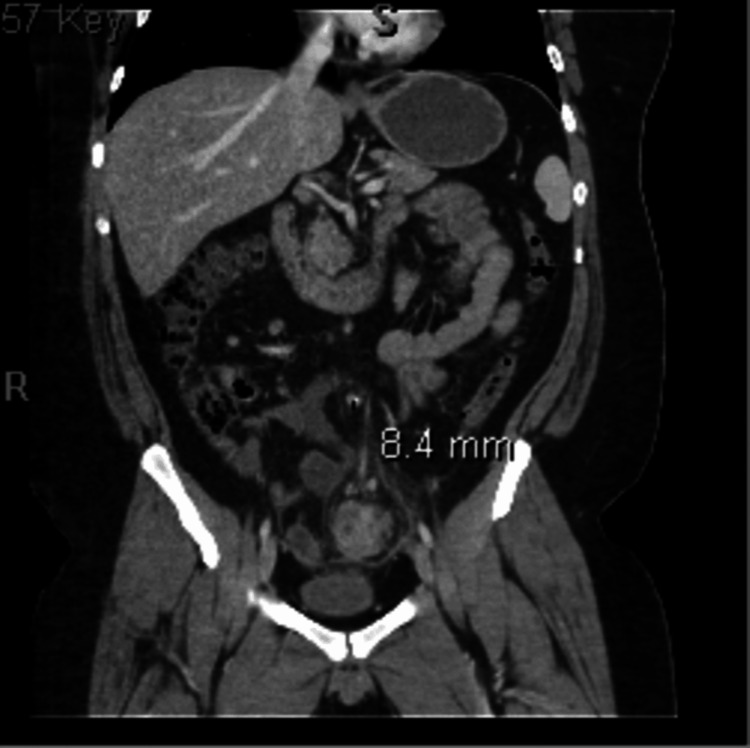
Coronal abdominal CT showing an area of inflammation near the diverticulum CT = computed tomography
